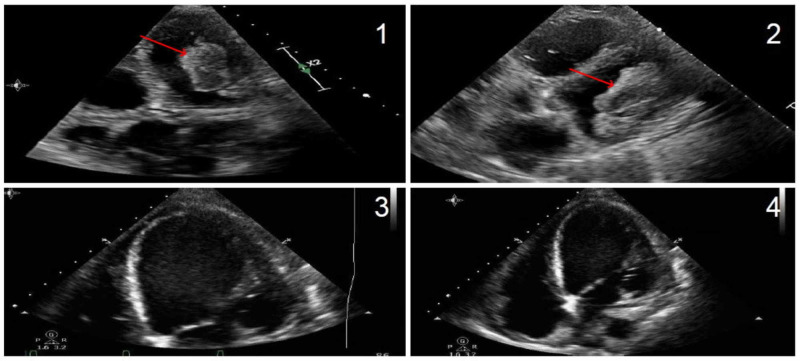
Echocardiogram demonstrating left ventricle thrombus Panels 1 and 2: Preoperative images (thrombus marked with arrows). Panels 3 and 4: Postthrombectomy images.
